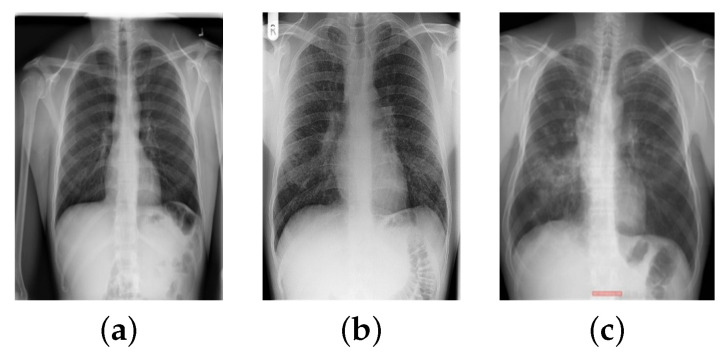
Different classes of chest X-ray images: (a) normal, (b) sarcoidosis-infected, (c) TB-infected.
